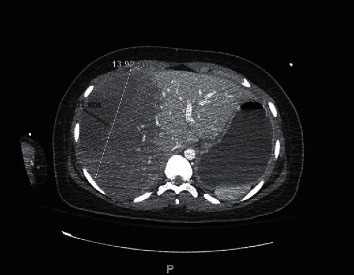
CTA abdomen on admission showing a large right hepatic subcapsular hematoma measuring 14 cm × 3.6 cm in size with active arterial extravasation.
